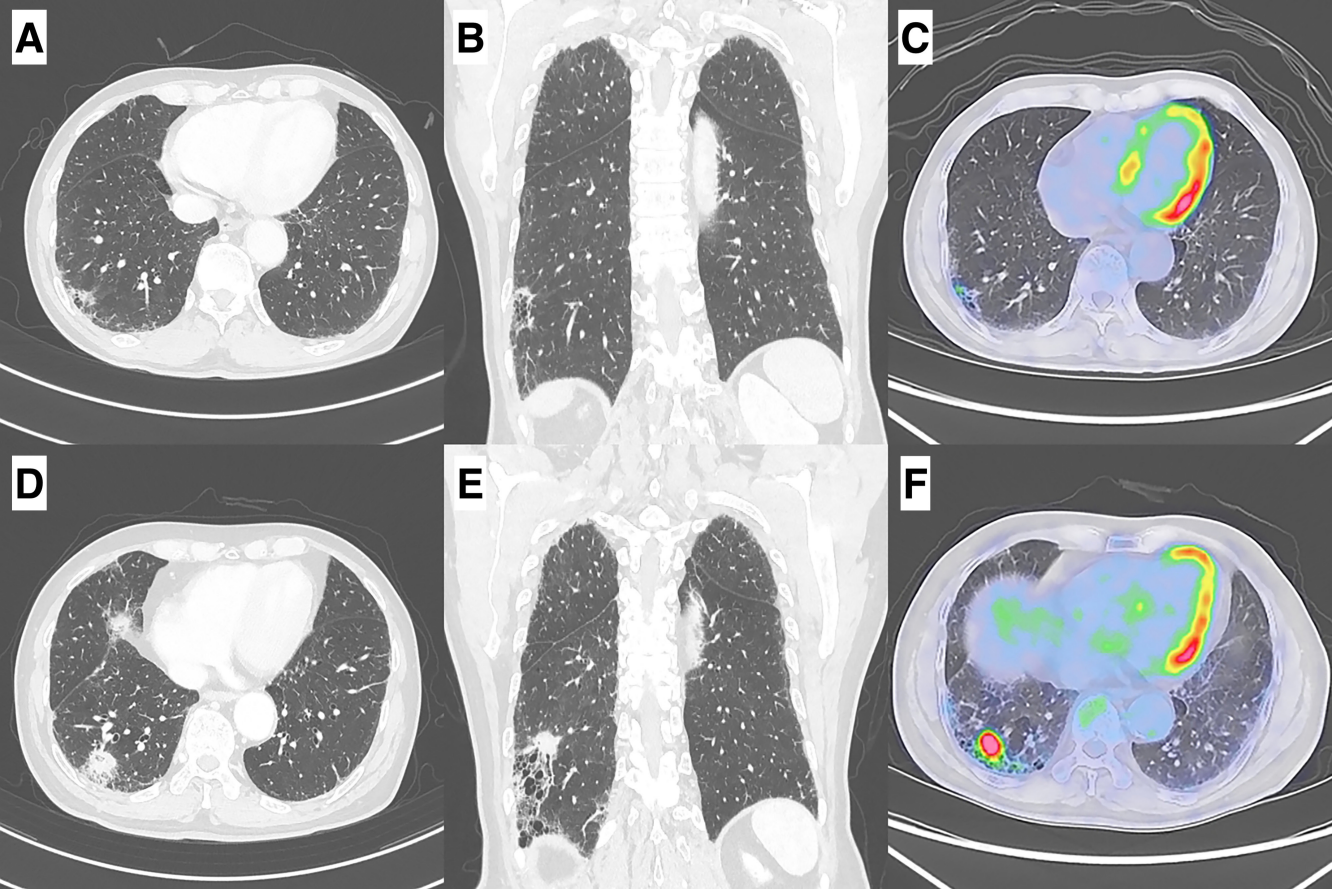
Chest CT and PET/CT findings. (A, B) Chest CT showed a 5 mm solitary nodule in the lateral basal segment of right lung at 9 years before surgery. (C) PET/CT showed an increased ^18^F‐fluorodeoxyglucose (FDG) uptake in the lesion (maximum standardised uptake value [SUVmax] = 2.8) at 9 years before surgery. (D, E) Chest CT revealed the lesion had grown to 3.2 cm immediately before surgery. (F) PET/CT revealed an increased FDG uptake (SUVmax = 8.4) in the lesion immediately before surgery.
